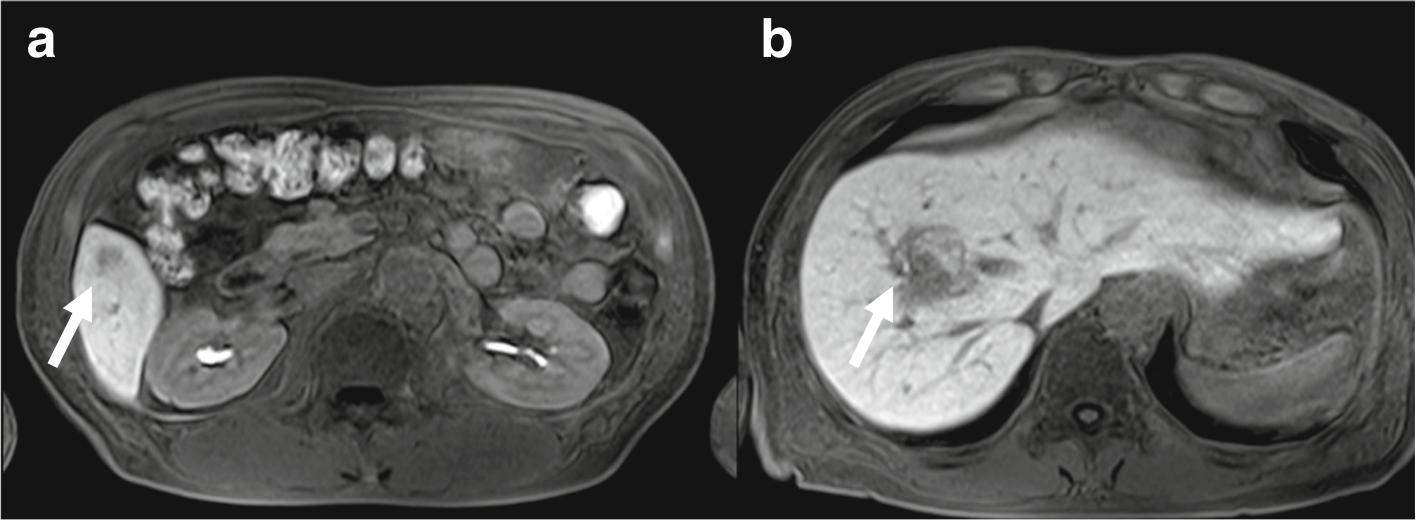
RI findings enhanced by Gadolinium-ethoxybenzyl-diethylenetriamine pentaacetic acid before surgery. Enhanced MRI revealed two tumors (arrows) located in a segment 8 and b segment 5
